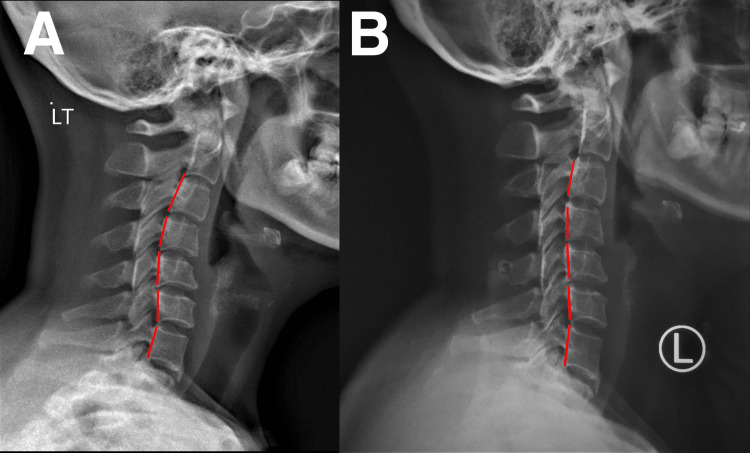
Cervical radiographs before and after therapy. A) Pre-treatment. Cervical deformity of reversed cervical lordosis at C1-4; B) Post-treatment. Cervical lordosis was reduced at C1-4.
